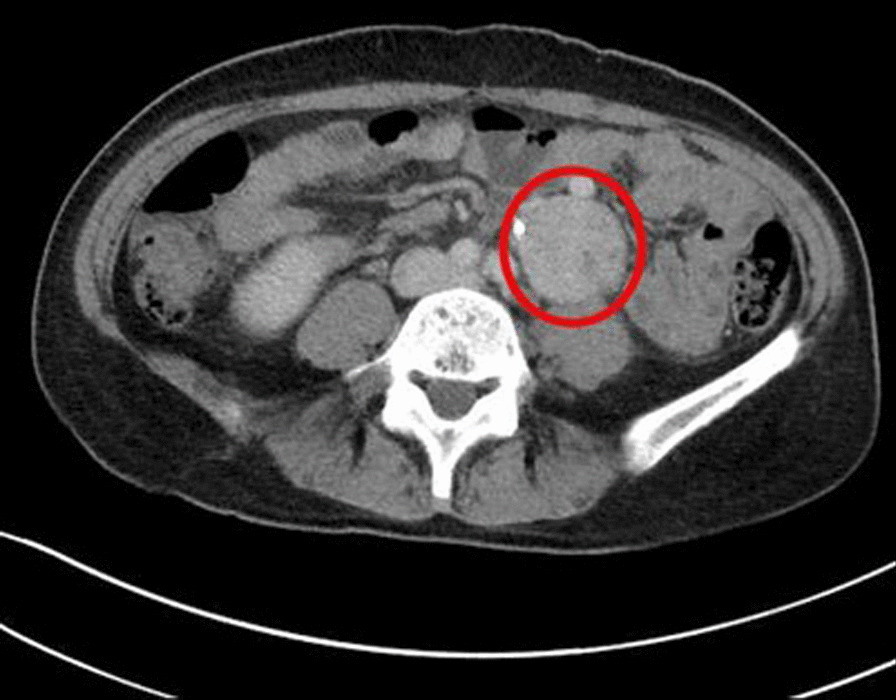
A round shape, up to 4.3 cm heterogenous enhanced mass over left retroperitoneum next to left ureter which is stented by double J tube in transverse view of contrast abdominal CT
